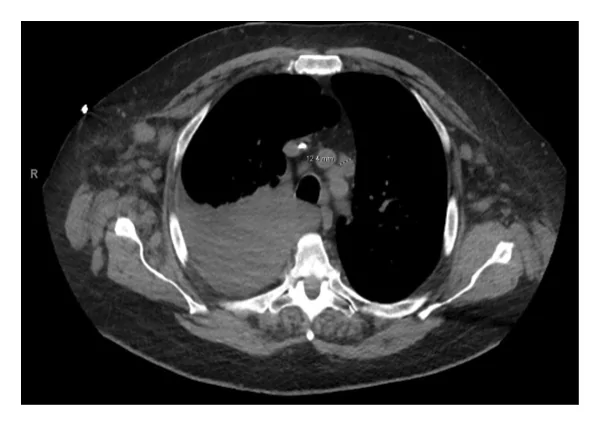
Computed tomography (CT) of the chest demonstrating a right‐sided pleural effusion associated with lymphoplasmacytic lymphoma in Patient B.
